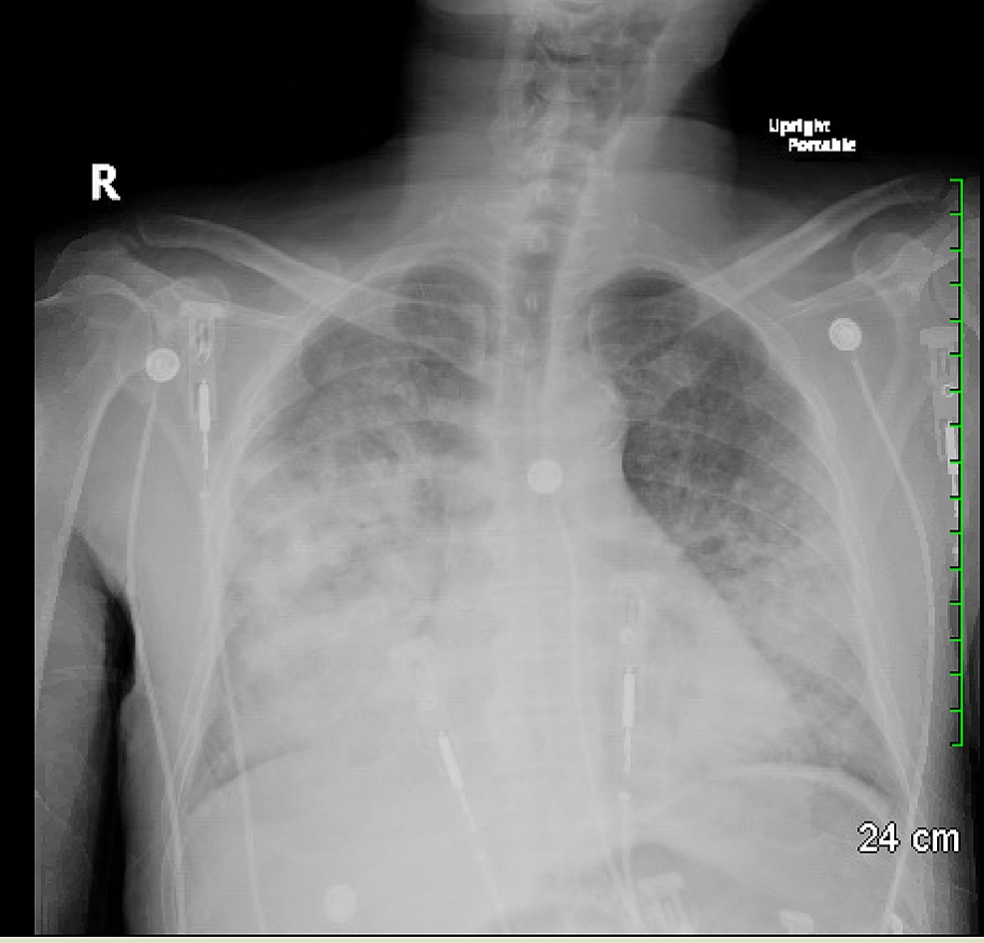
Chest X-ray showing confluent opacities in the lungs bilaterally (right greater than left)
